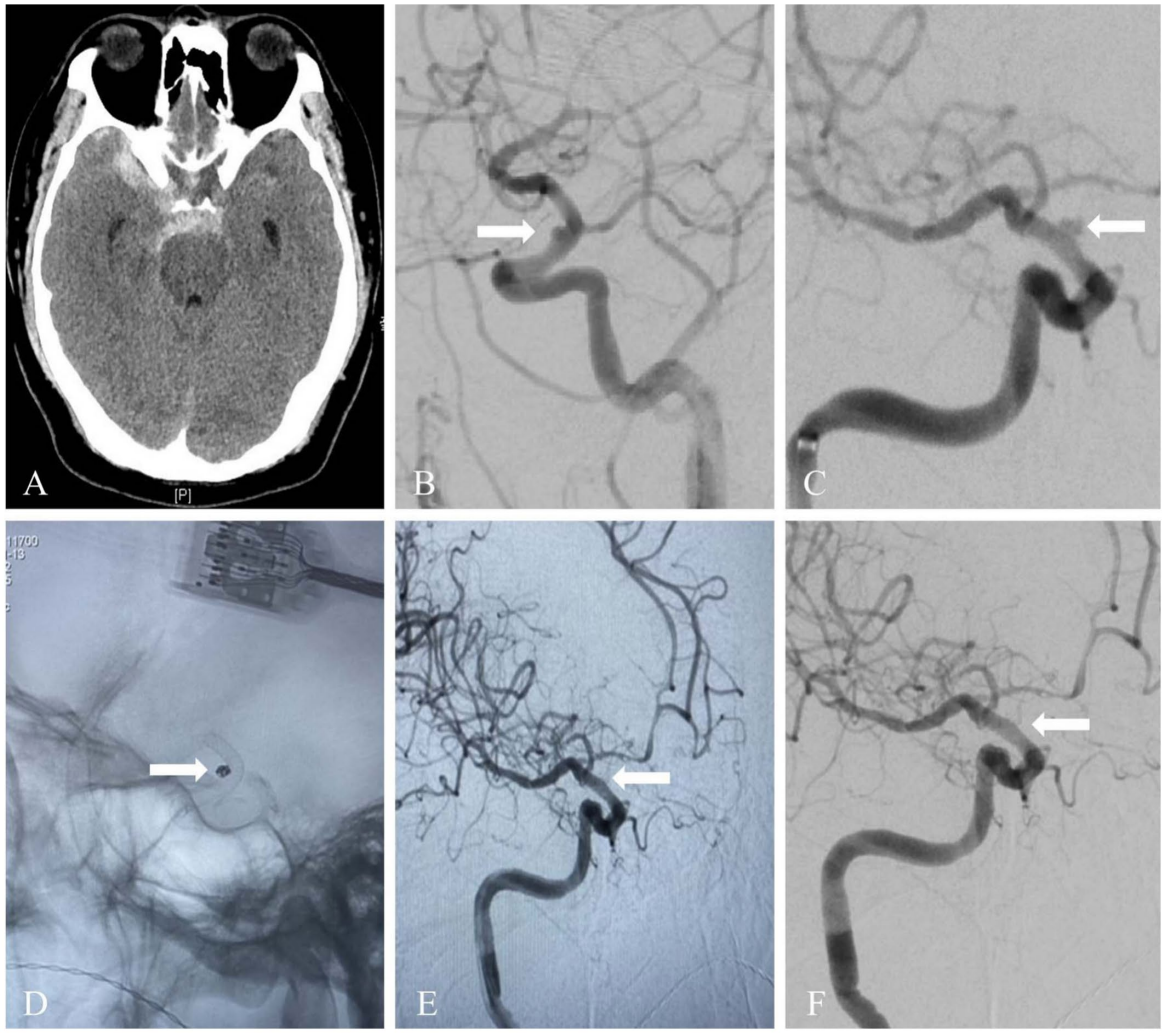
A ruptured blood blister aneurysm was treated with PED and adjunctive coiling. (A) Head computed tomography image of a 51-year-old patient showed acute subarachnoid hemorrhage. (B,C) Diagnostic cerebral angiography revealed a blood blister aneurysm located on the supraclinoid segment of the right internal carotid artery (white arrow). (D) The aneurysm was treated with PED and adjunctive coiling. Fluoroscopic image showed the flow diverter and coil mass (white arrow). (E) Immediate angiography after PED deployment showed complete occlusion (white arrow). (F) Follow-up angiography at 10 months showed complete occlusion of the aneurysm (white arrow).
